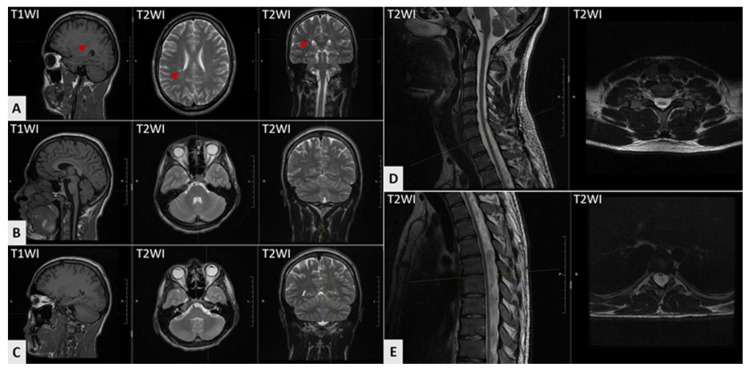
Brain and spinal cord MRI findings of the proband at ages 18 and 20 years, respectively. (A) A focus of increased signal intensity is visible on T2-weighted images in the right parietal lobe (arrows). (B,C) No evidence of cerebral or cerebellar atrophy is present. The brainstem appears normal without pathological changes. (D,E) Spinal cord MRI demonstrates generalized atrophy in both the cervical (D) and thoracic (E) regions.
